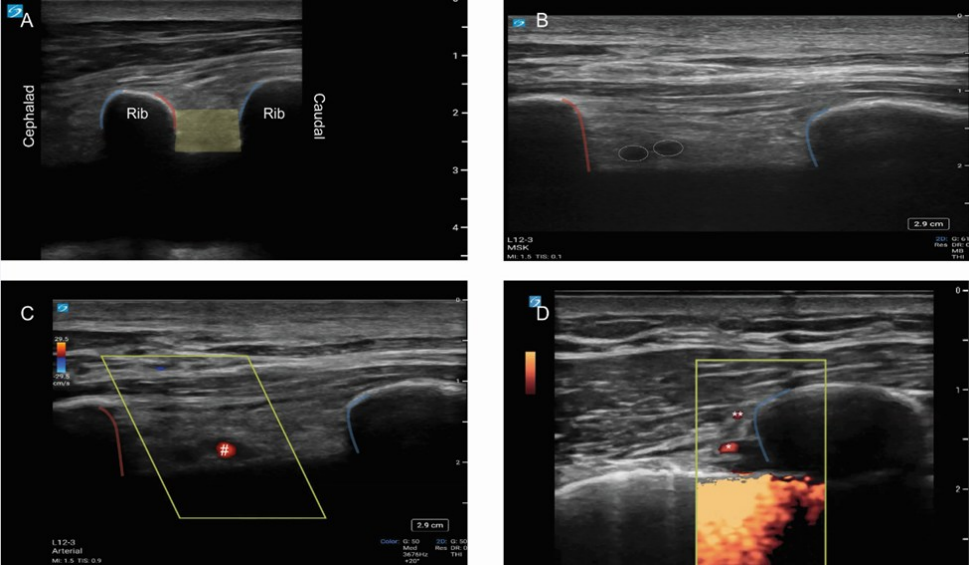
A) Identified superior (blue outline) and inferior (red outline) borders of the rib along with the intercostal space (shaded yellow). B) Rounded hypoechoic structures beneath the rib (intercostal artery (ICA) & intercostal vein). C) ICA identified by color Doppler (red). D) Power Doppler.
